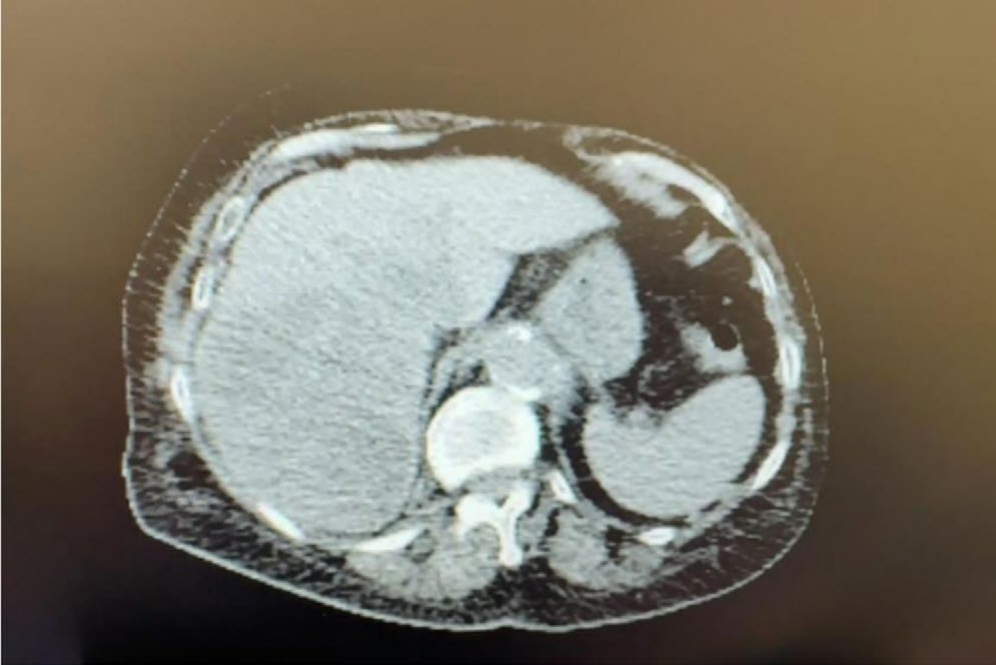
Computed tomography revealed the presence of necrotic liver lesions.
